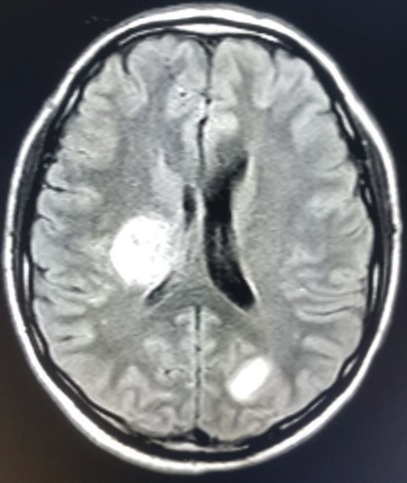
Magnetic resonance image of dorsolumber spine
